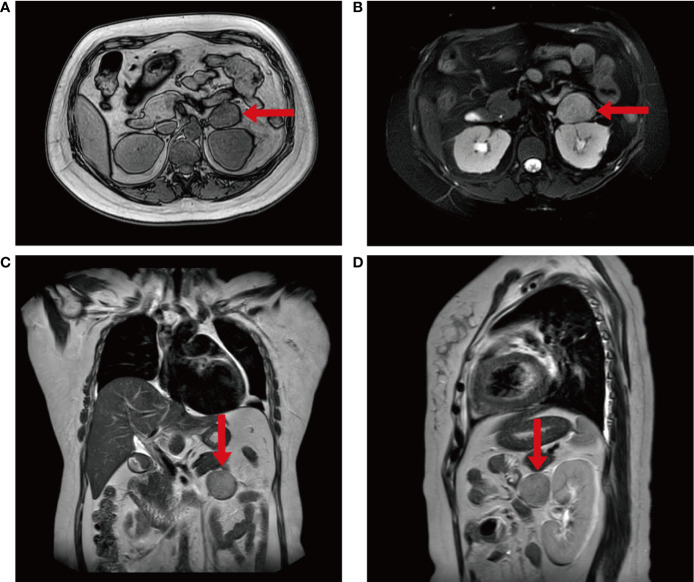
The imaging findings of the patient. (A–D) Plain MRI scan of the patient’s abdomen showed an abnormal signal mass in front of the left kidney, which was about 4.7x3.3x3.7cm in size and had a clear boundary.
